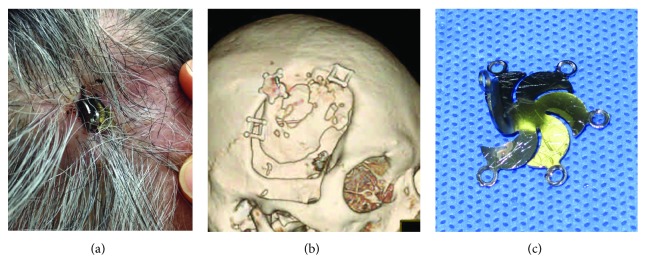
Change in shape of a pinwheel-shaped titanium mini plate. (a) A titanium plate that had been inserted during cranioplasty 16 years previously is protruding from the scalp through a circular defect. (b) Three-dimensional computed tomography (3D-CT) scan image showing that part of the titanium plate has been bent upwards. (c) After surgical removal, the bend in the plate is clearly visible.
